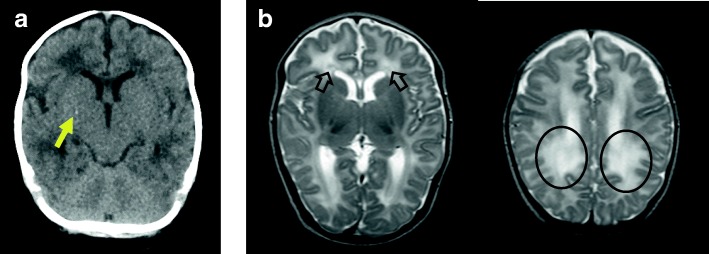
CT and MRI findings. a Head CT scan at 1.5 months of age. The arrow indicates intracranial calcification. b Axial T2-weighted brain MRI at 1.5 months of age showing diffuse abnormalities of the white matter in the parietal, occipital, and frontal lobes (circle) as well as periventricular cysts (arrows) around the posterior horn of the bilateral lateral ventricles. No polymicrogyria, lissencephaly, or ventriculomegaly is observed
